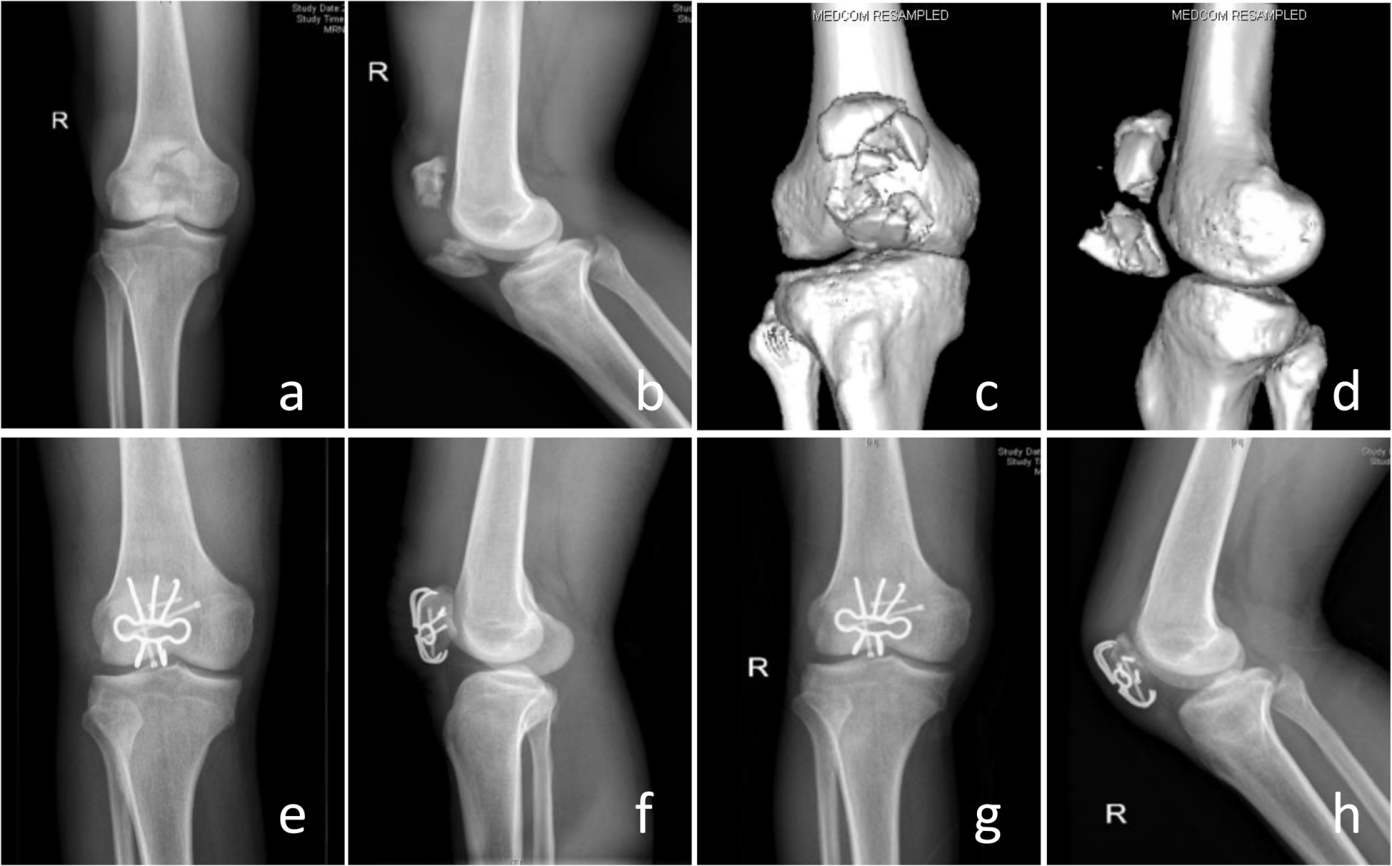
Comminuted fracture (AO/OTA 34C3) of the patella in a 48-year-old male patient after a car accident (a-d). Open reduction and internal fixation with TNSMPC and compression screws were performed. X-rays immediately after surgery (e, f) and 3 months later (g, h) showed desirable outcomes
